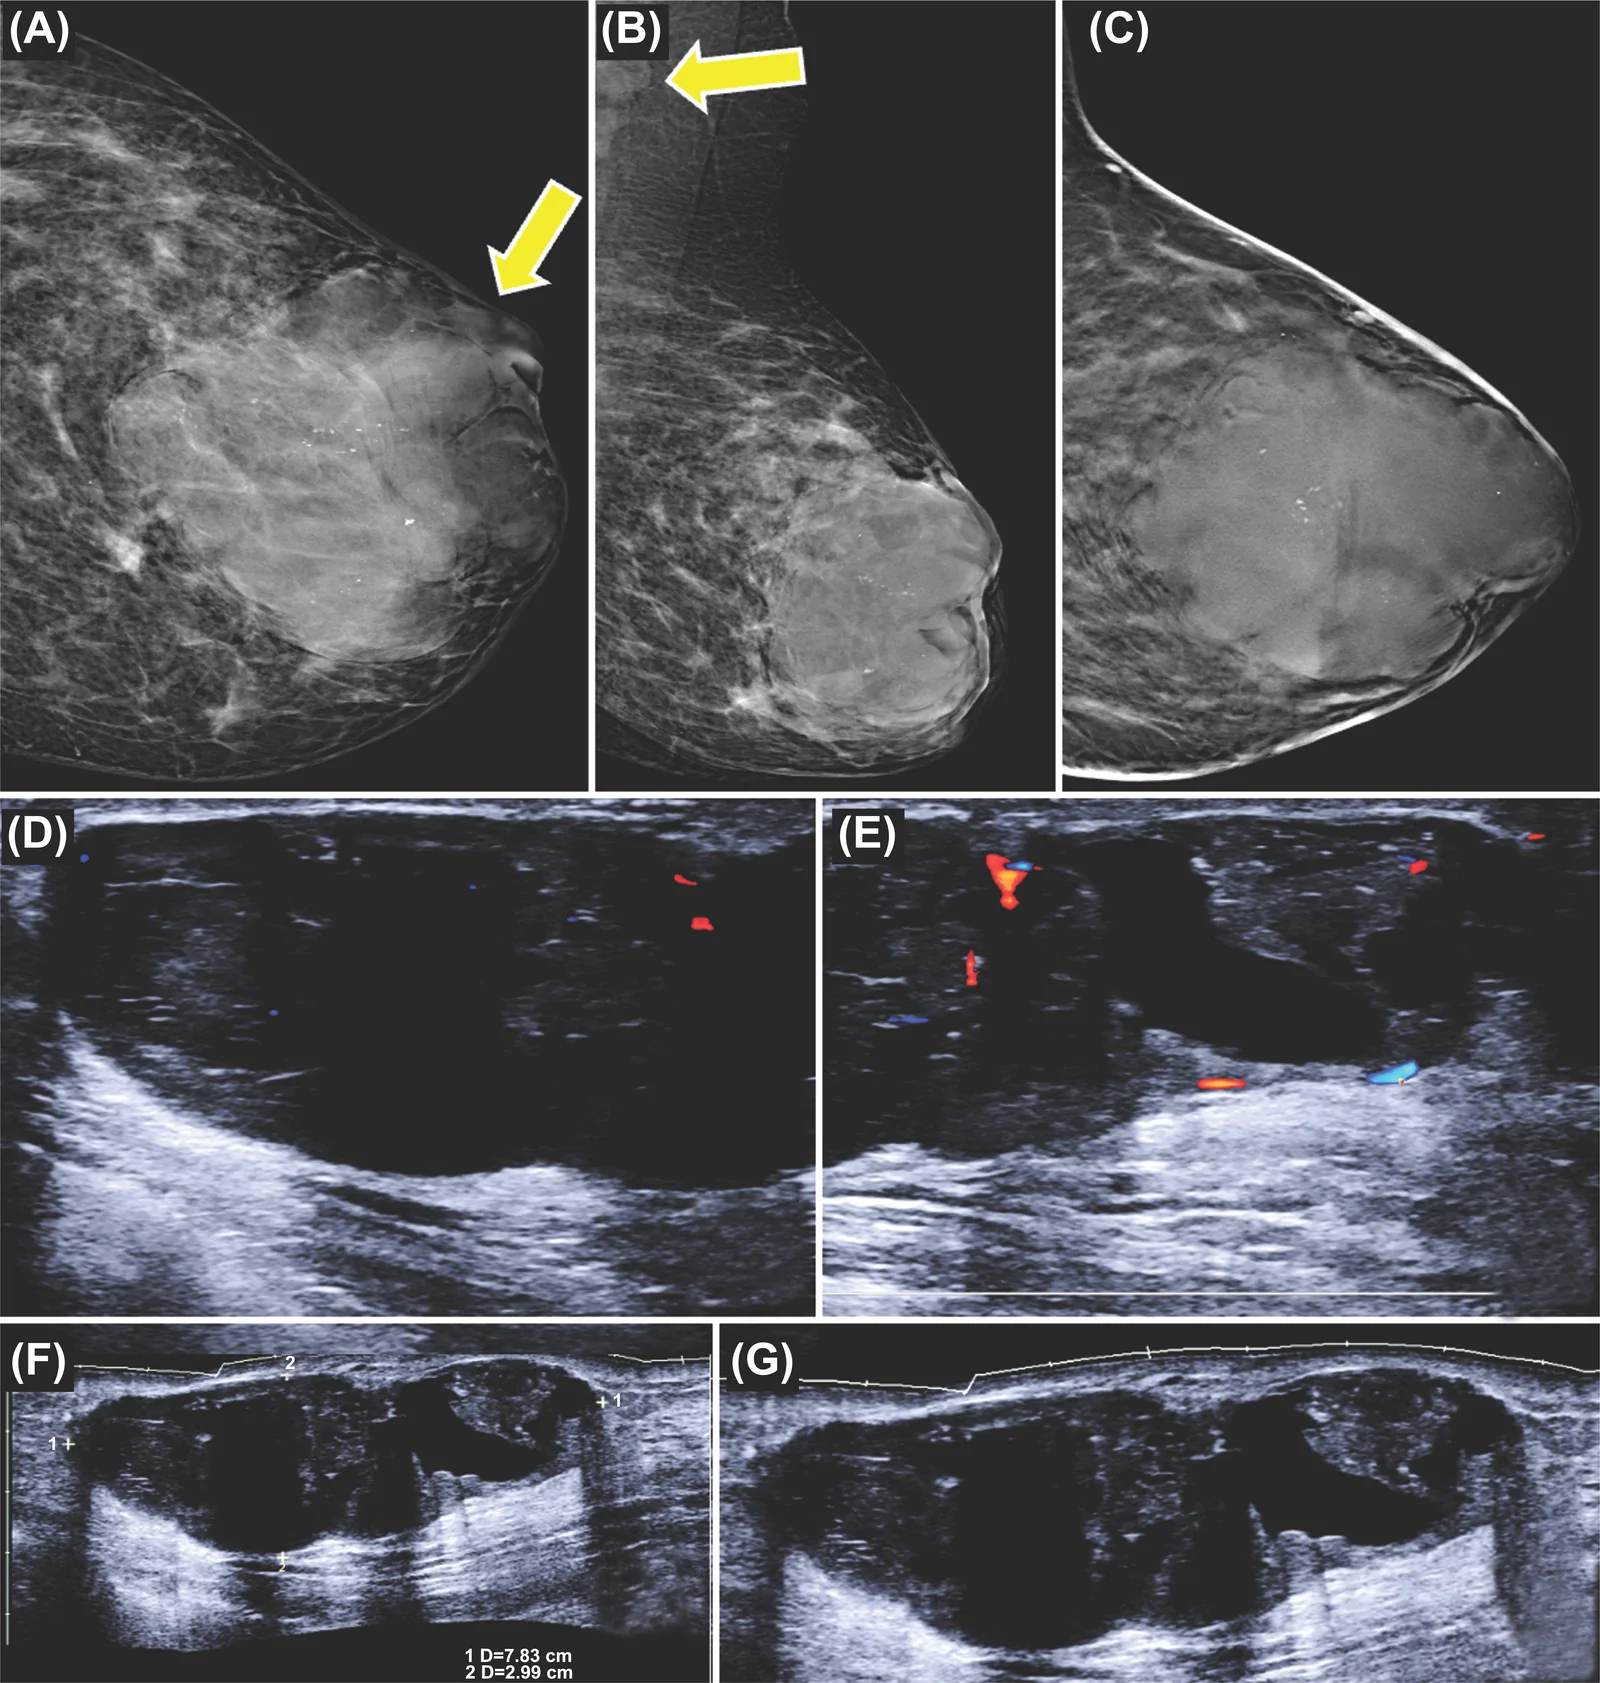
BI-RADS 4A lesion. Craniocaudal mammographic view (A) of the left breast shows an oval, circumscribed nodule with similar density to surrounding tissue, associated with heterogeneous calcifications and cutaneous extension, located centrally in the anterior-middle third (yellow arrow). Medio-lateral oblique view (B) shows preserved axillary lymph nodes (yellow arrow). Tomosynthesis (C) highlights architectural distortion more clearly. US images (D, E) reveal a complex, solid-cystic, oval mass parallel to the skin, with central and peripheral Doppler flow. The mass measures approximately 78.3 × 29.9 mm in its longest axes (F), and additional grayscale imaging confirms its morphology (G).
